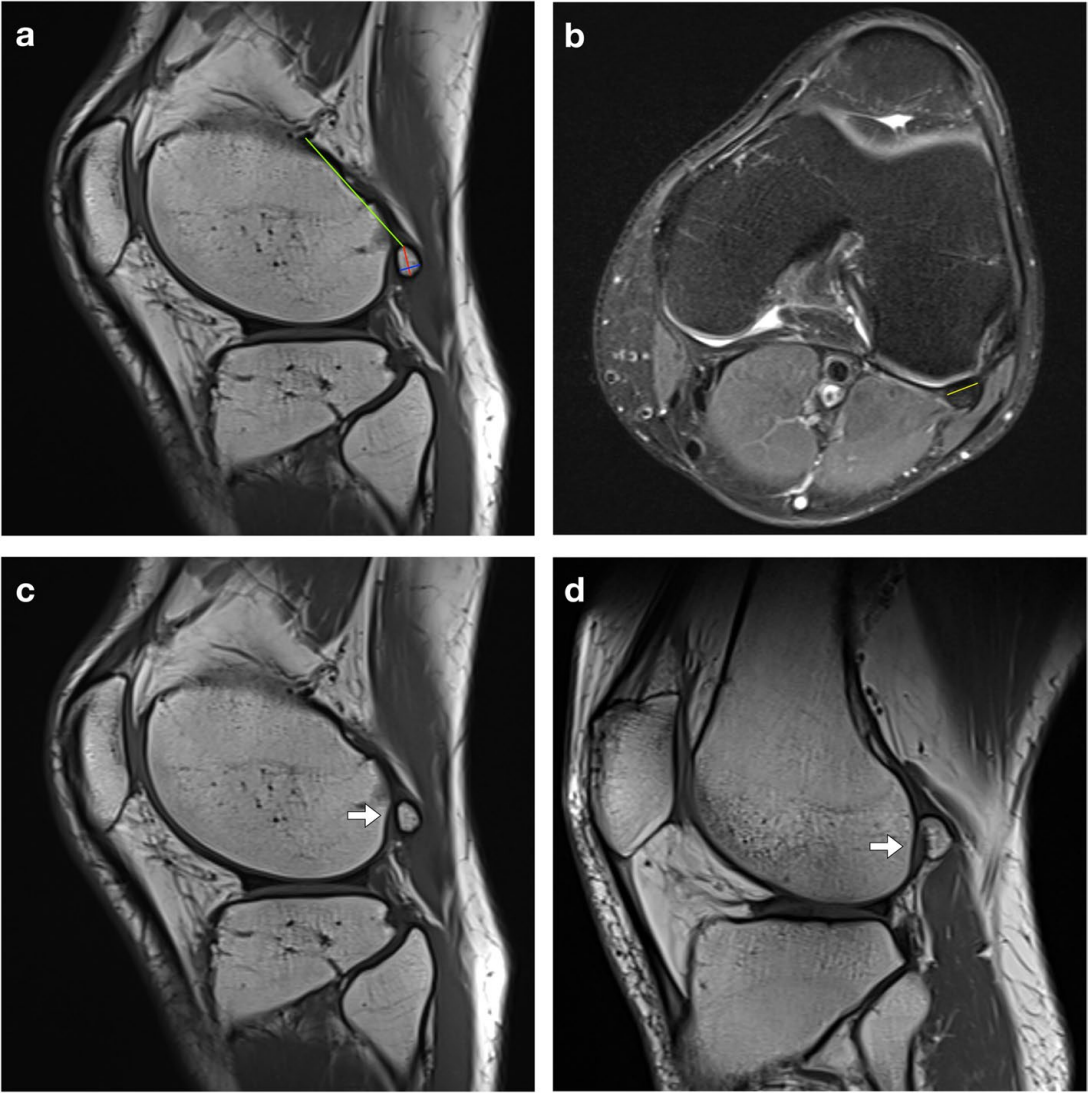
Magnetic resonance imaging (MRI) measurement and articulating groove. a Sagittal MRI of the knee shows the measurement of the maximum length (red line), thickness (blue line) of fabella, and the distance between the fabella and the insertion of the lateral head of the gastrocnemius onto the femur (green line); b Axial MRI of the knee shows the measurement of the maximum width of the fabella (yellow line); MRI of the knee show (c) concave and (d) flat contours of the articulating groove of the lateral femoral condyle of the femur (arrow)
